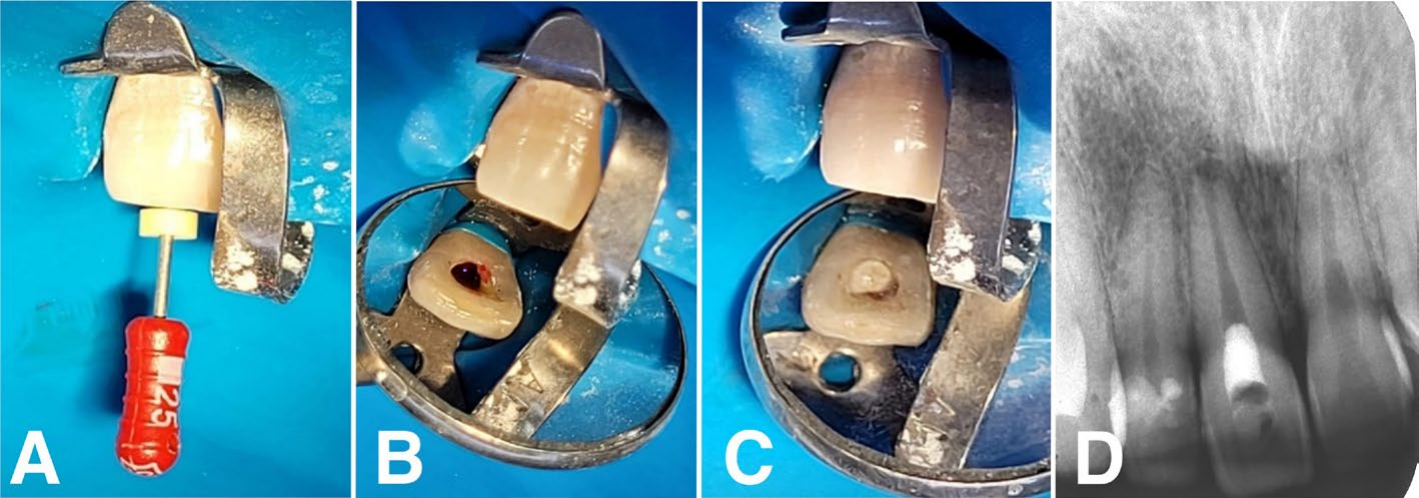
(A) A #25 K‐File introduced in the canal 2 mm beyond the root canal length to promote bleeding; (B) Intracanal bleeding and blood clot formation at the level of the cementoenamel junction (CEJ); (C) Clinical and (D) radiographic images of bioceramic material placed over the blood clot, forming a 3 mm plug.
